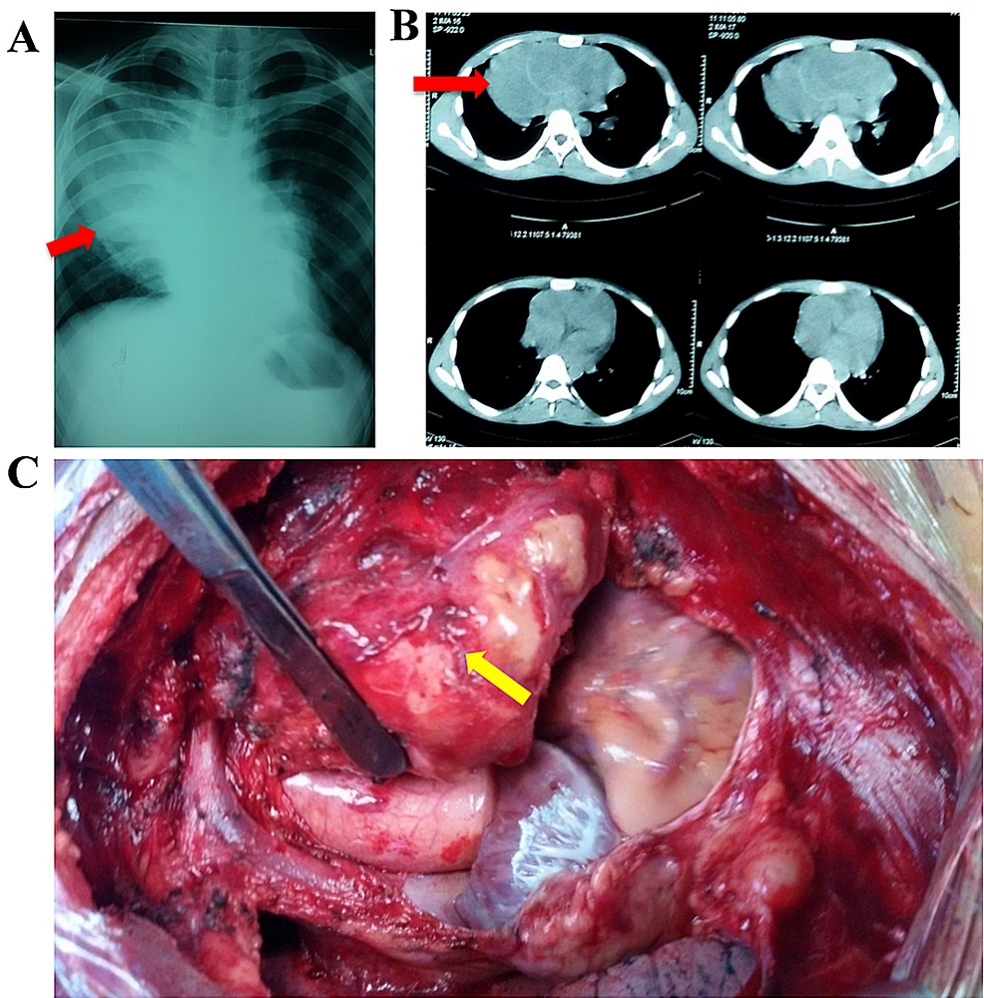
(A) Chest X-ray; (B) contrast-enhanced CT scan; (C) intraoperative picture. Red and yellow arrows represent malignant fibrosarcoma in radiological and intraoperative figures, respectively. CT: computed tomography
